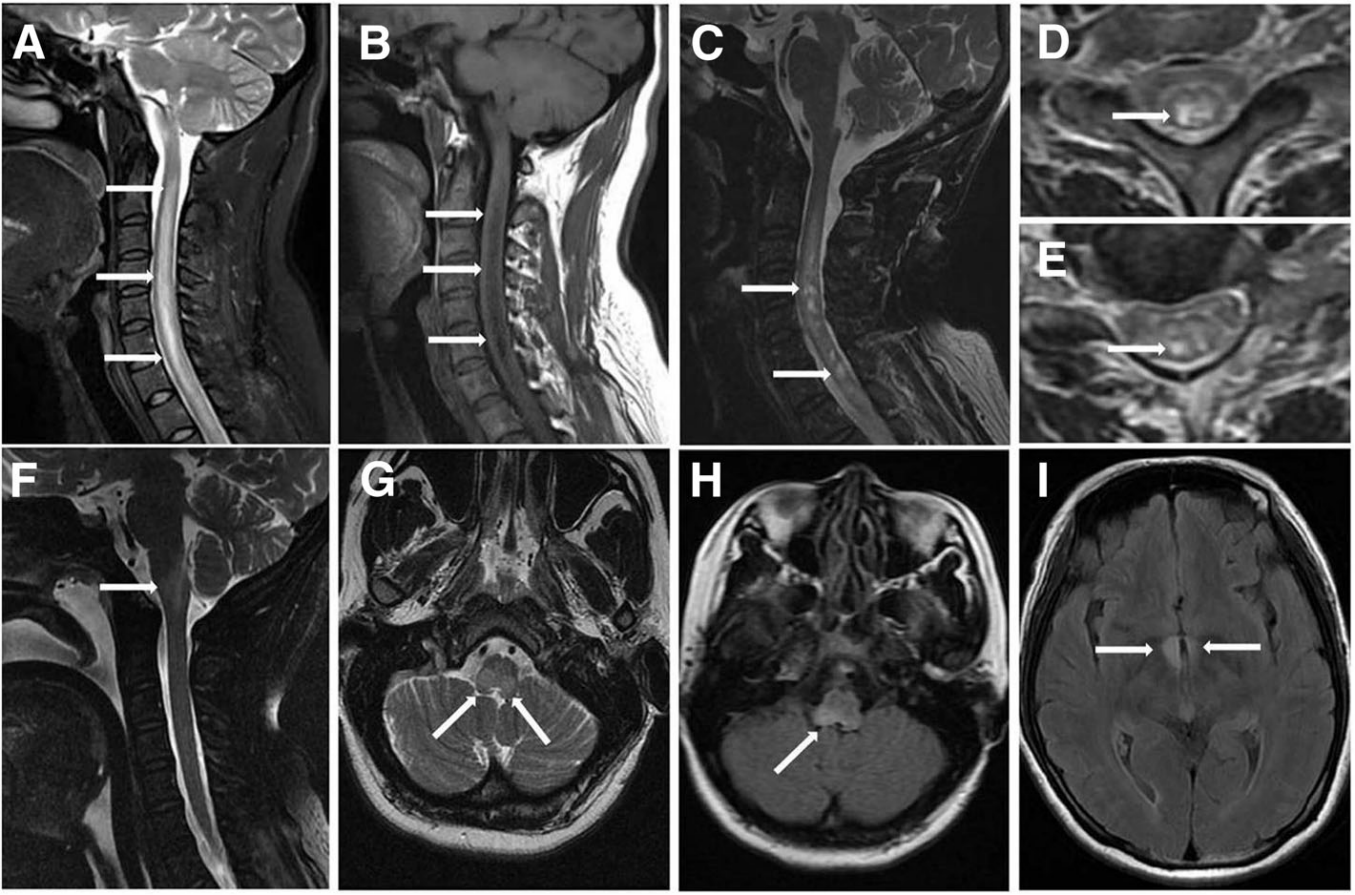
Representative MRI abnormalities (arrows) in patients with NMOSD with CTD. a and b are from a 35-year-old woman with NMOSD and SS; (a) shows longitudinally extensive transverse myelitis (LETM) lesions on T2WI, and b shows ‘T1 dark’ associated with LETM. c, d and e, from a 40-year-old woman with NMOSD and SLE, show bright spotty lesions (BSLs) associated with LETM on T2WI. f, from a 38-year-old woman with NMOSD and SS, shows an area postrema lesion on T2WI. g, A 45-year-old woman with RA, shows a medulla oblongata lesion on T2WI. h, from a 39-year-old woman with NMOSD and undifferentiated CTD (UCTD), shows an area postrema lesion on FLAIR imaging. i, A 45-year-old woman with NMOSD and UCTD, showed bilateral hypothalamus lesions on the FLAIR imaging
